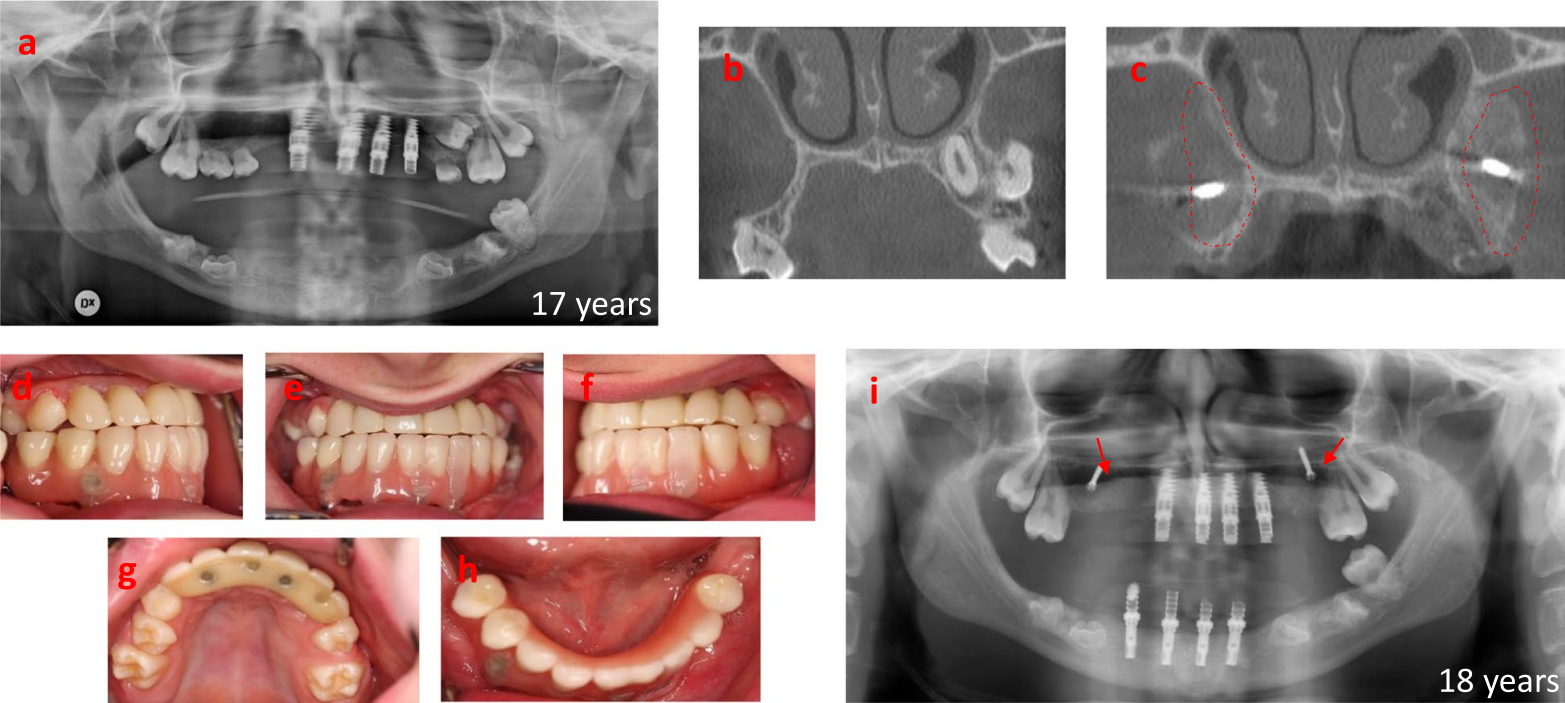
Phase 2 of the treatment at the age of 17 years. After successful healing after extraction of frontal teeth in the upper jaw and successful osseointegration of the first four implants, the teeth that were in the way for implant placement in the frontal part of the lower jaw were surgically removed. a Six months Post-extraction-panoramic radiograph at age of 17. Four titanium implants, Branemark System Mk III, TiUnite, RP, were placed in positions 32–42 with two stage surgery. b CBCT of the posterior parts of upper jaw showing a very narrow and thin bone volume. After extraction of the remaining premolars in the upper jaw and healing for three months, reconstruction of the width of the alveolar process was performed bilaterally with c free autologous crista iliaca block graft as highlighted in new CBCT by red lines in. d-h Photographs overviews of both fixed Polymethyl methacrylate (PMMA) temporary bridges, made on four implants in each jaw. i Radiograph panoramic overview at the age of 18 years of the fixed PMMA-bridges with temporary abutments. Notice the fixations screws of the bone grafts in the upper jaw (arrows)
